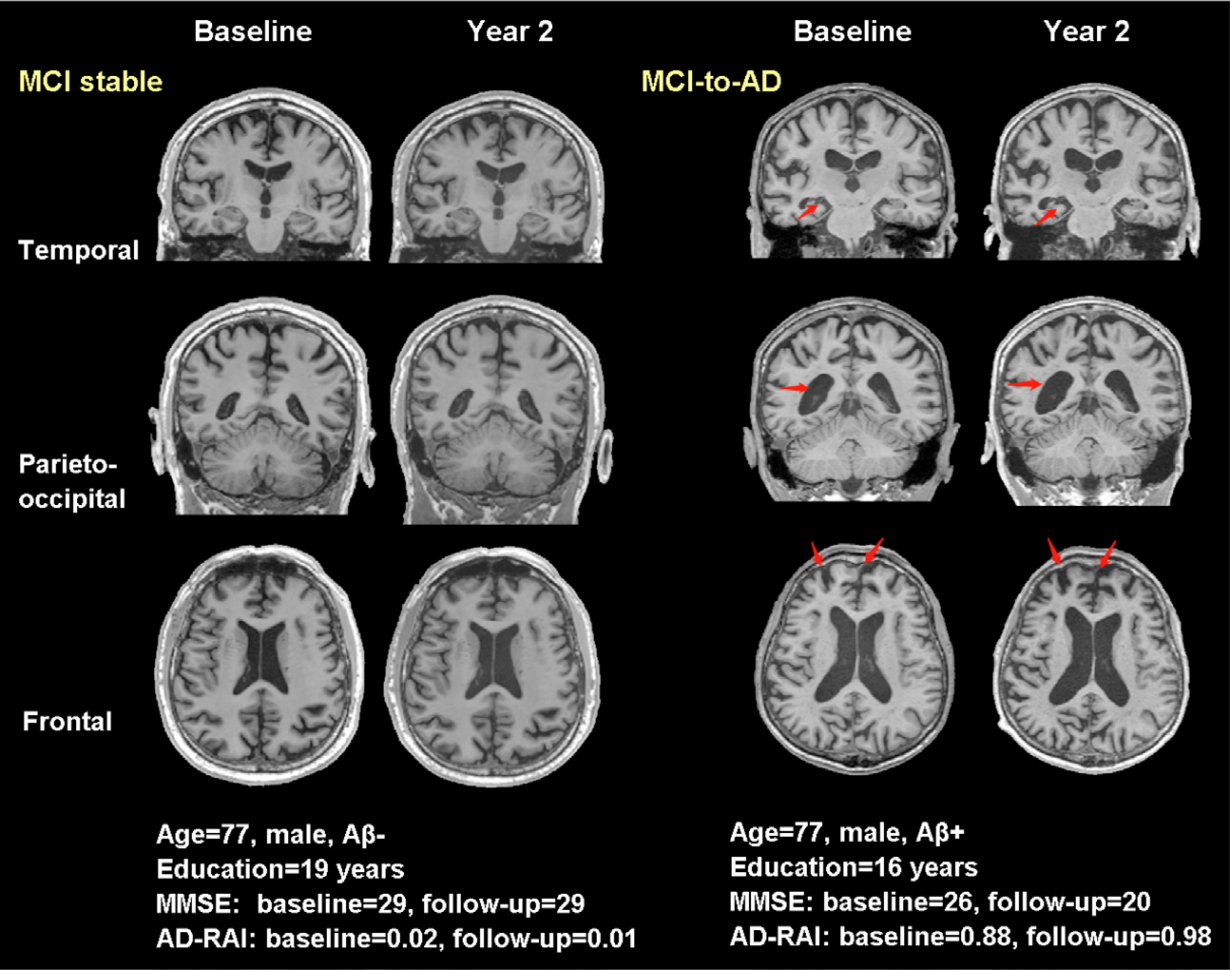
Typical real cases of MCI stable and MCI-to-AD subjects. T1-weighted (T1W) images at baseline and two years were shown for the two typical cases in temporal, parieto-occipital and frontal view. Red arrows pointed to the regions with significant atrophy by comparing the T1W images of the same subject over two years. The typical case of MCI stable did not present progressed atrophy during the two years, while the case of MCI-to-AD showed increased width of right choroid fissure and temporal horn (temporal view), enlargement of lateral ventricle (parieto-occipital view) and increased frontal lobe atrophy (frontal view). Aβ-: CSF-based Aβ_42_ >192 pg/ml at baseline and 2 years; Aβ+: CSF-based Aβ_42_ <192 pg/ml at baseline and 2 years; AD-RAI: AD resemblance atrophy index.
